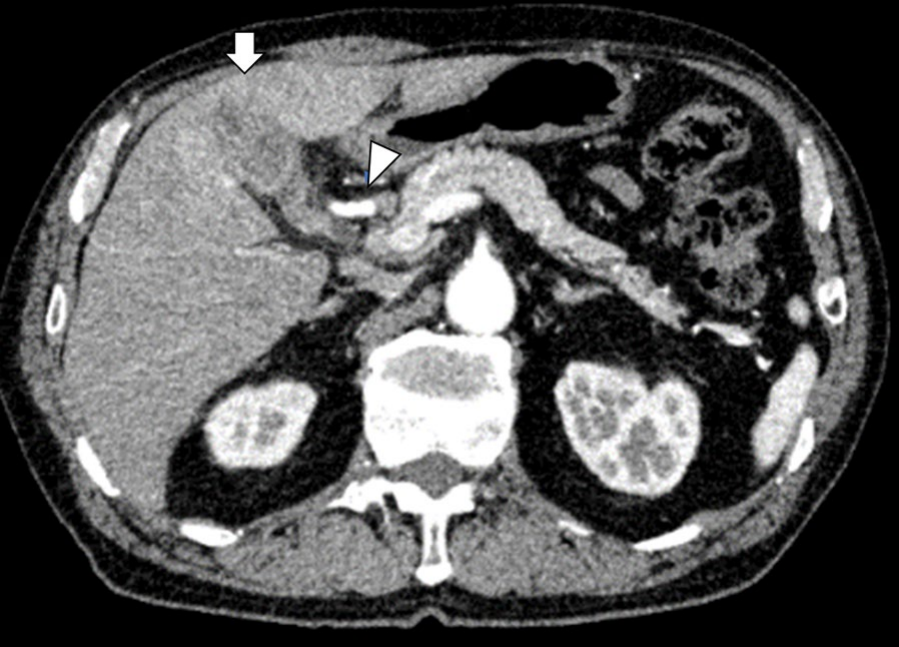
Preoperative enhanced CT imaging of case 1. The arrow indicates the main tumor. The arrowhead indicates the right hepatic artery (RHA). A tumor of the gallbladder infiltrated to the liver parenchyma and spread to the cystic and common hepatic duct with contact of RHA
